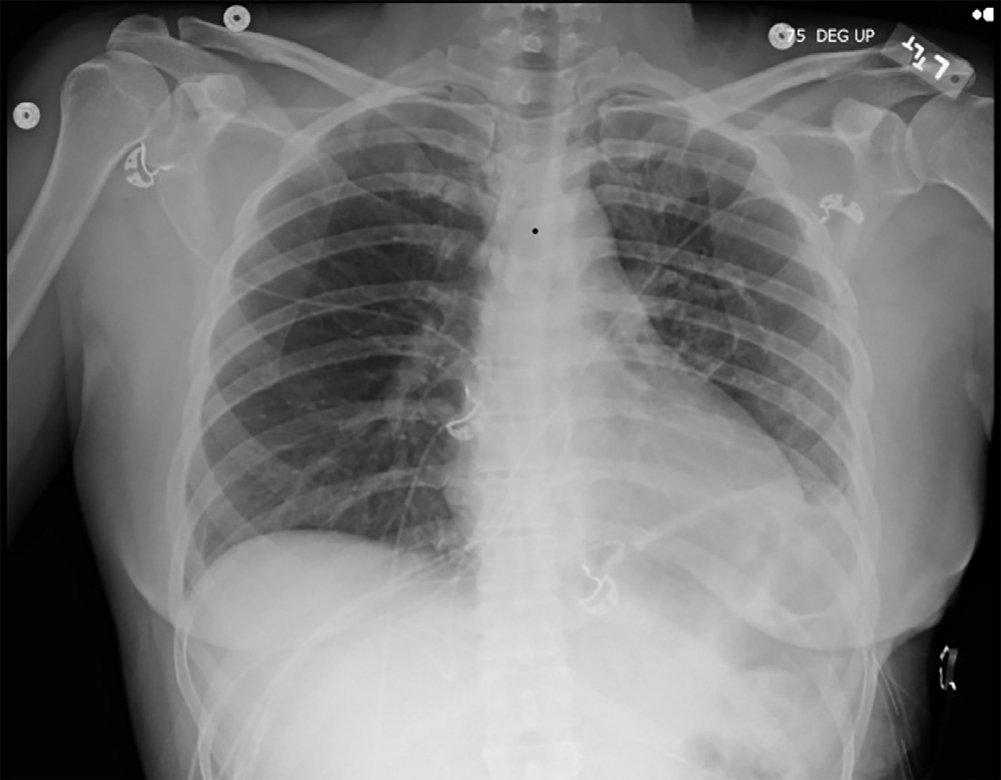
Post-VATS pleurodesis chest X-ray demonstrating normal lung volume, mild patchy atelectasis in the left lung, no significant pleural effusion or pneumothorax, and stable cardio-mediastinal and skeletal structures. The imaging confirms lung re-expansion and postoperative stability.
